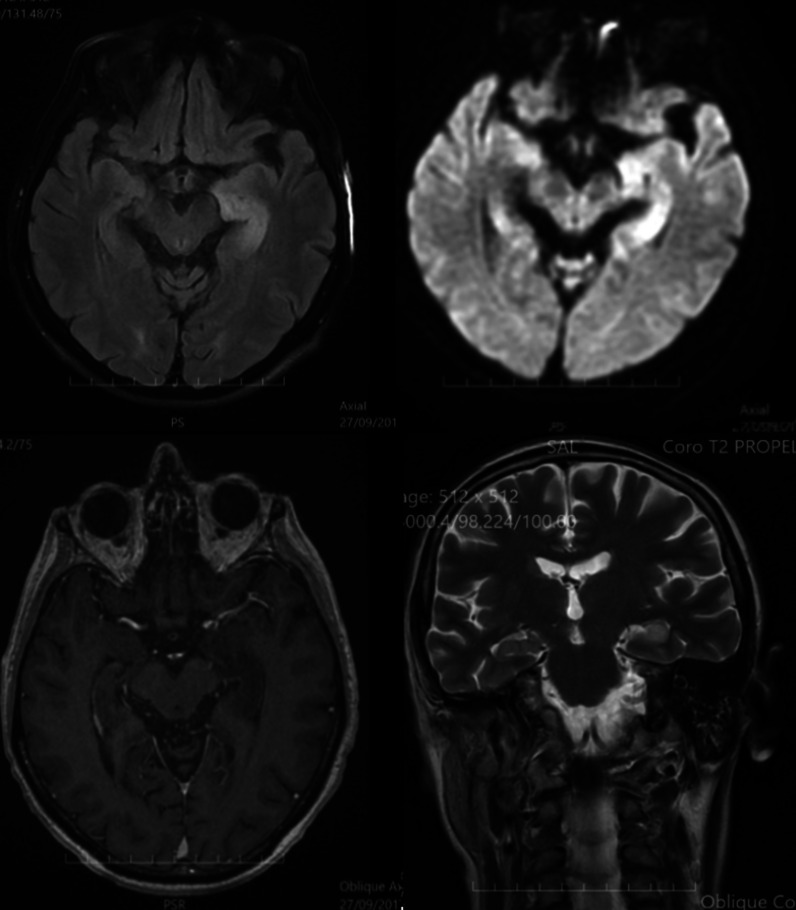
MRI: left hippocampal and parahippocampal hypersignal, without contrast enhancement
